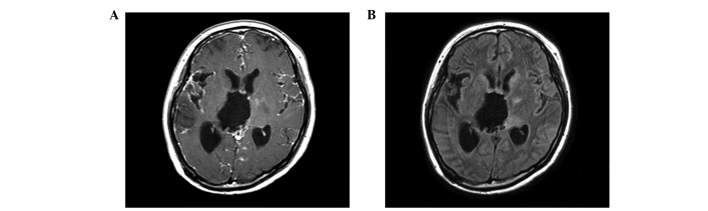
MRI showed progression of leptomeningeal dissemination following four cycles of TMZ and five cycles of bevacizumab from the beginning of recurrent therapy. (A) T1-weighted contrast-enhanced MRI; (B) fluid-attenuated inversion recovery imaging. MRI, magnetic resonance imaging; TMZ, temozolamide.
